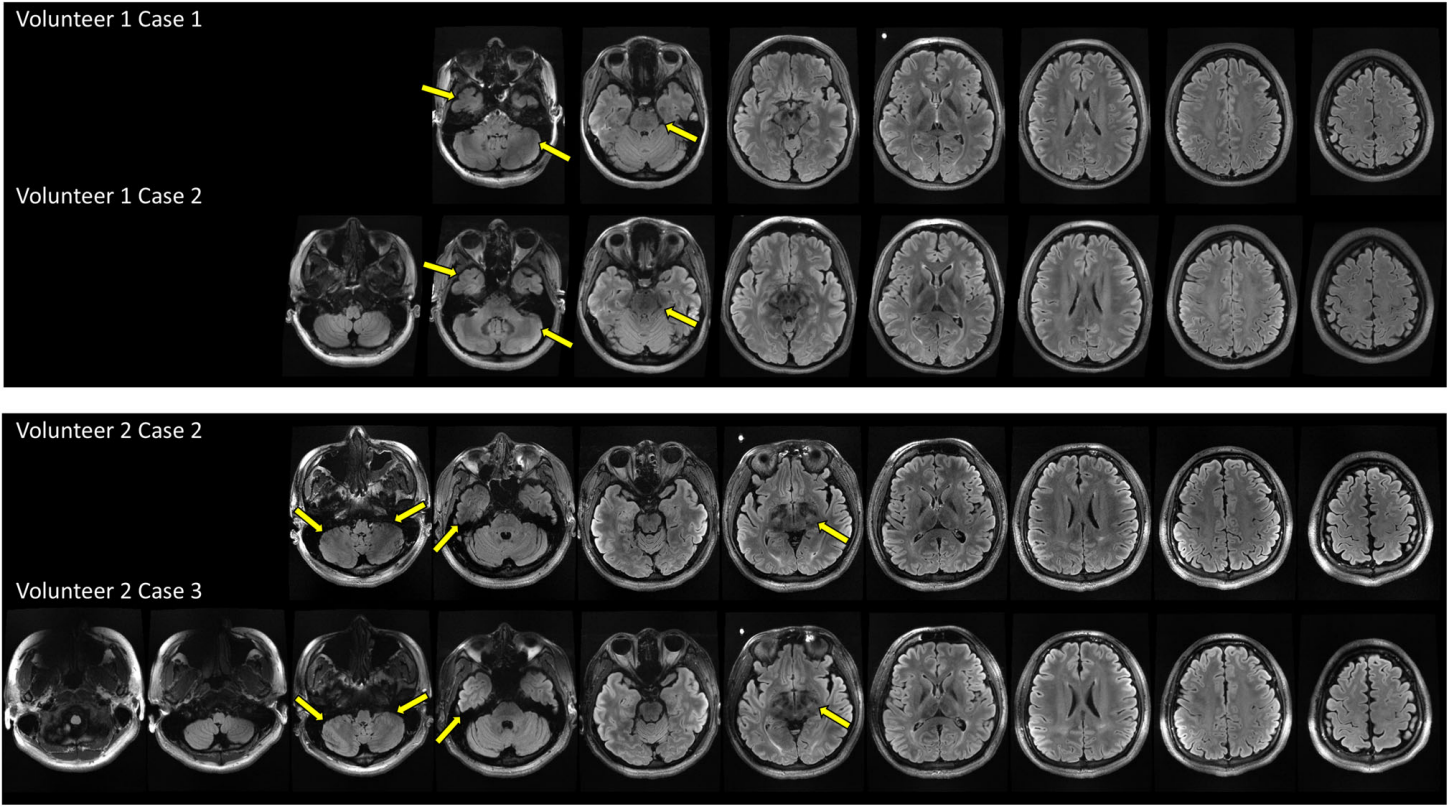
T_2_‐FLAIR images (raw from the scanner) acquired on volunteers 1 and 2 using the 60‐channel Tx/32‐channel Rx Tic Tac Toe RF coil system. The sequence used for Volunteer 1 was 64 slices, 0.85 × 0.85 × 1.7 mm resolution. The sequence used for Volunteer 2 was 0.75 × 0.75 × 1.5 mm resolution; Case 2 was 80 slices and Case 3 was 100 slices. Volunteer 1 has an intracranial volume (ICV) of 1.46 L, head dimensions of 19 cm AP, 16.2 cm RL, and 14.7 cm from the top of the skull to the bottom of the cerebellum. Volunteer 2 has an ICV of 1.67 L, head dimensions of 19.5 cm AP, 17.7 cm RL, and 15.2 cm from the top of the skull to the bottom of the cerebellum. Arrows show regions of improvement between the cases.
